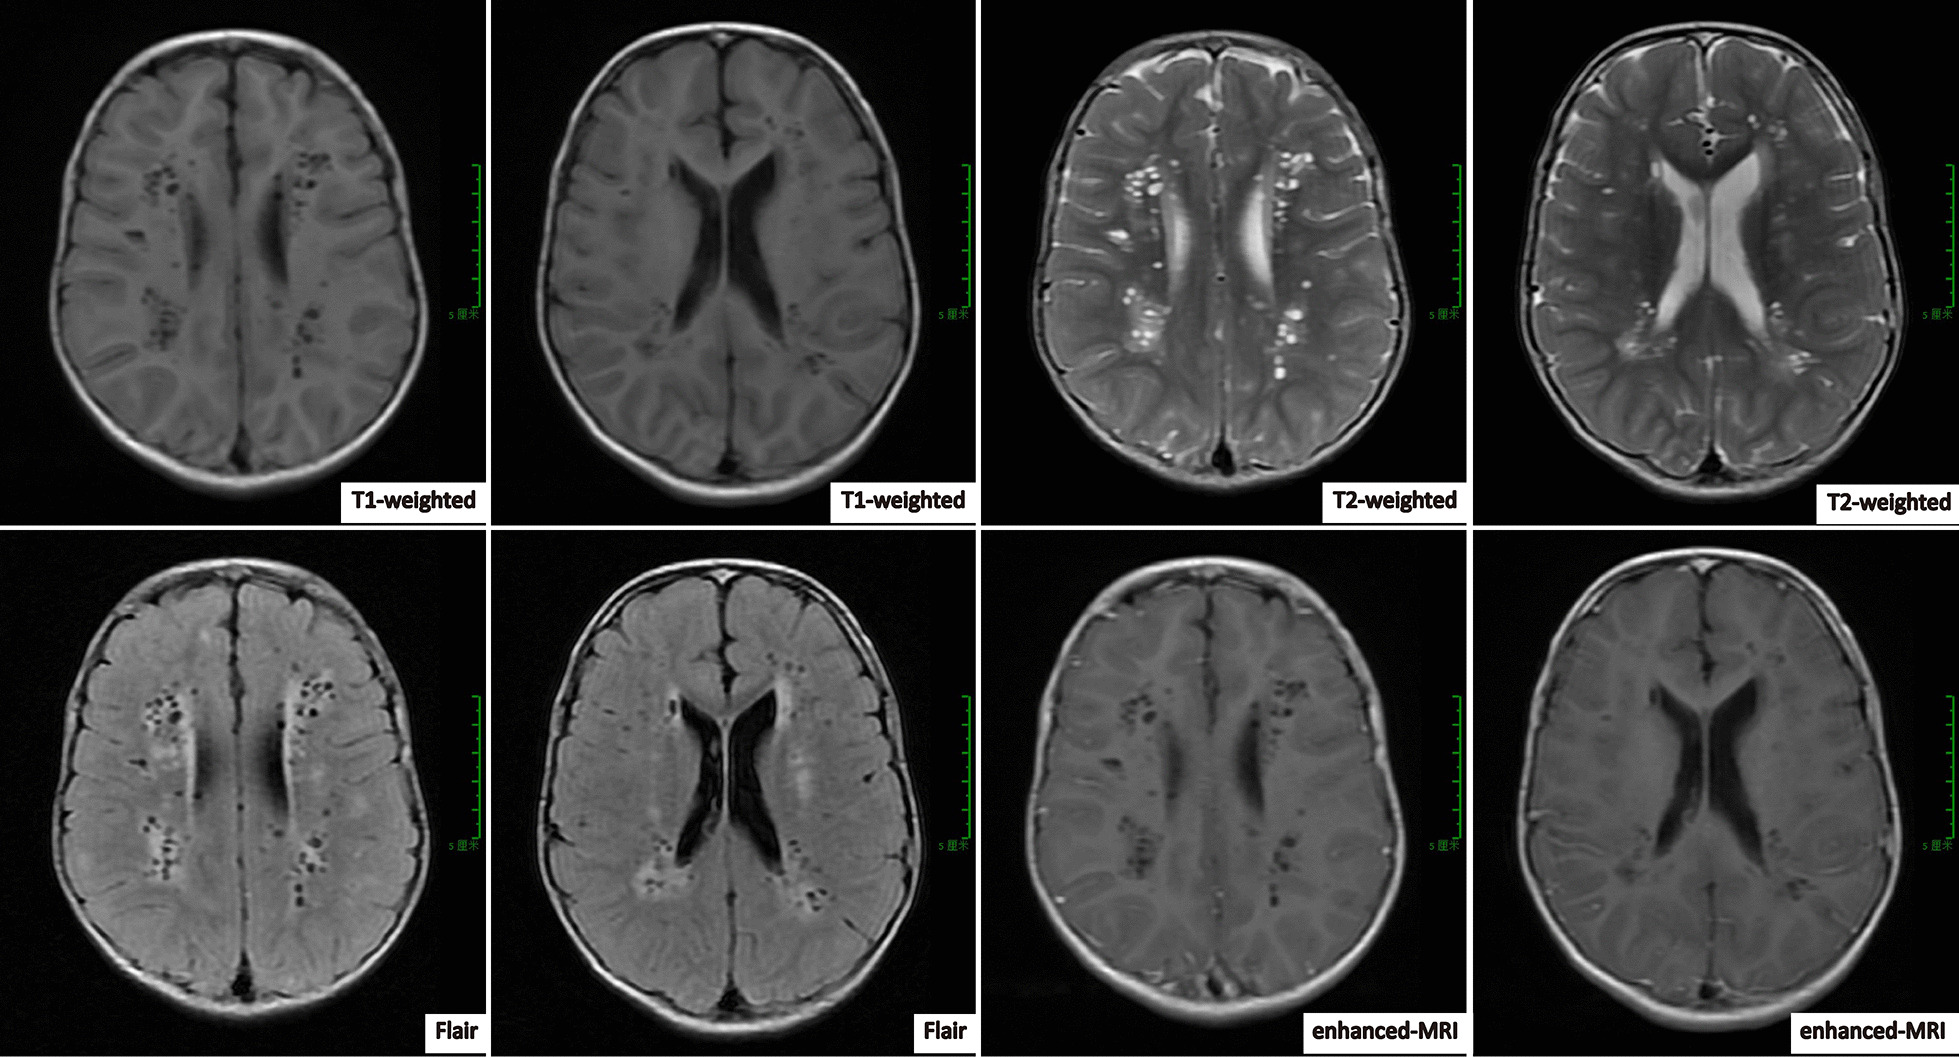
Brain MRI of patient 1 at the age of five years. Note the presence of ventriculomegaly, bilateral periventricular leukomalacia, and multiple gliotic lesions
